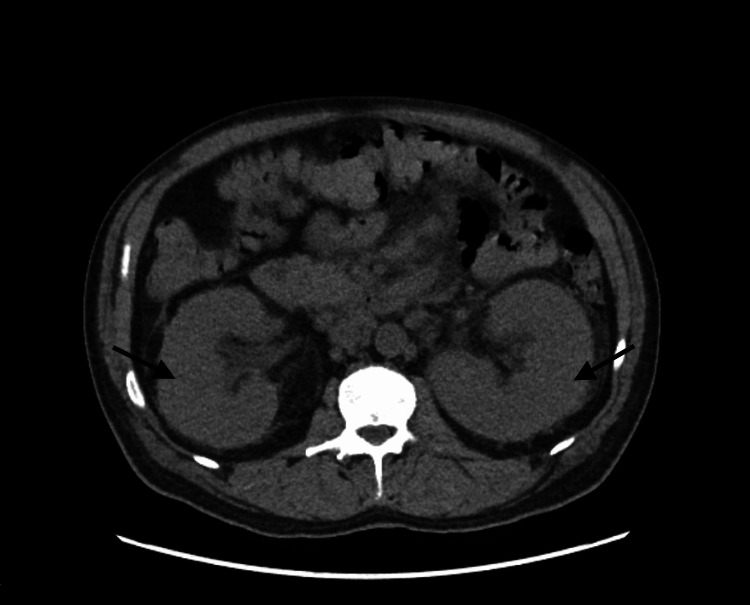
CT abdomen and pelvis without contrast (axial orientation) demonstrating severe bilateral nephromegaly measuring up to 20 cm indicated by arrows
